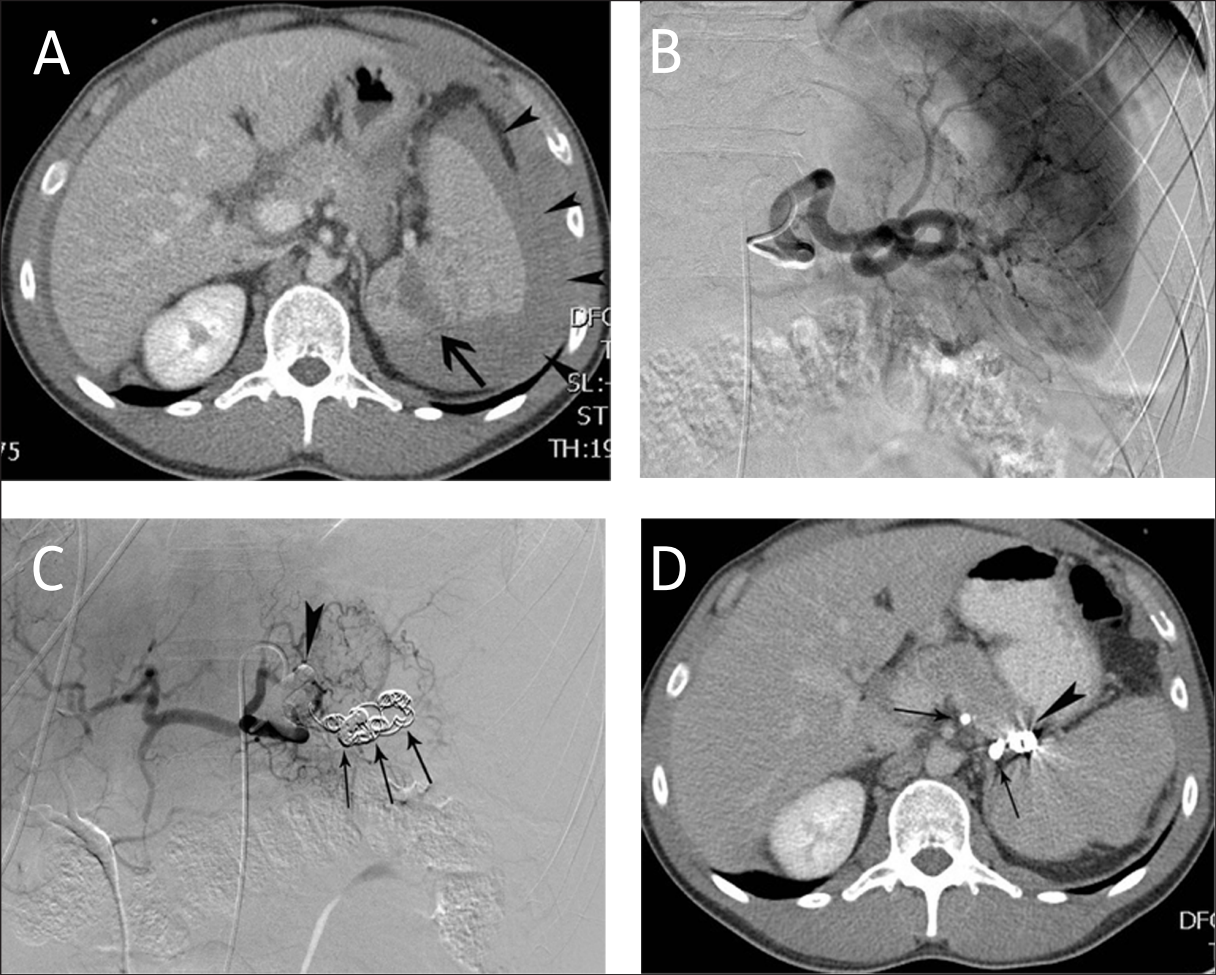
Main splenic artery embolization for trauma. (A) CT-scan in a 27-year-old man after a traffic accident revealed splenic laceration (arrow) and a perisplenic hematoma (arrowheads). The volume of the spleen was 270 ml. (B) Corresponding selective splenic angiography did not reveal contrast extravasation. (C) Main splenic artery embolization was performed with coils (arrows) and glue (arrowhead). (D) Follow-up CT-scan 8 months later demonstrated the glue (arrows) and coils (arrowhead) in the splenic artery. The total splenic volume was 290 ml.
